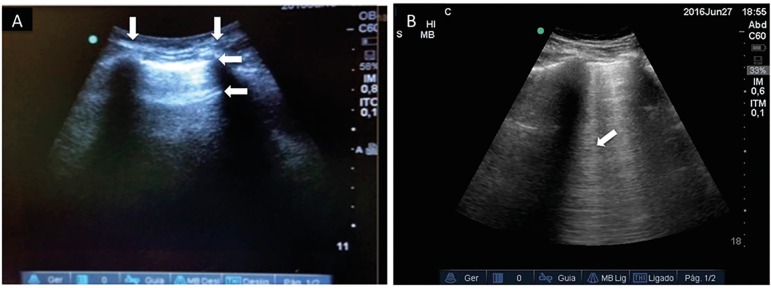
Lung ultrasound is largely based on the interpretation of artifacts
